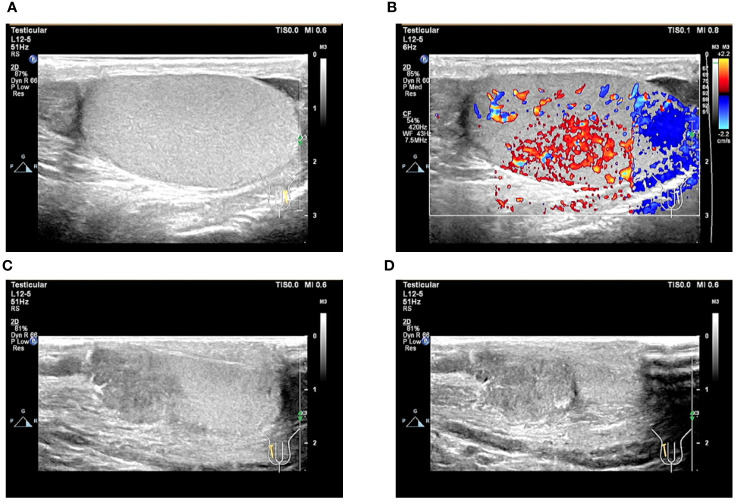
Ultrasound image of the testis. (A, B) Ultrasound image of the left testis; (C, D) Ultrasound image of the right testis.
